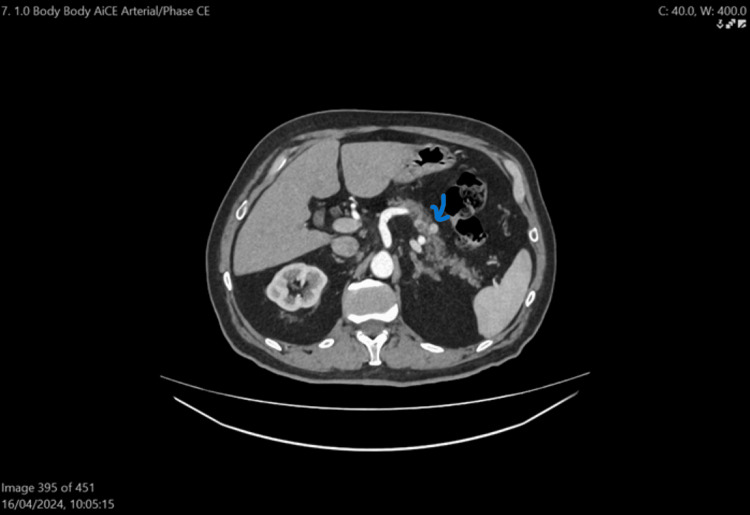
CT Neck Thorax Abdomen showing pancreatic nodule Arrow showing pancreatic nodule.
